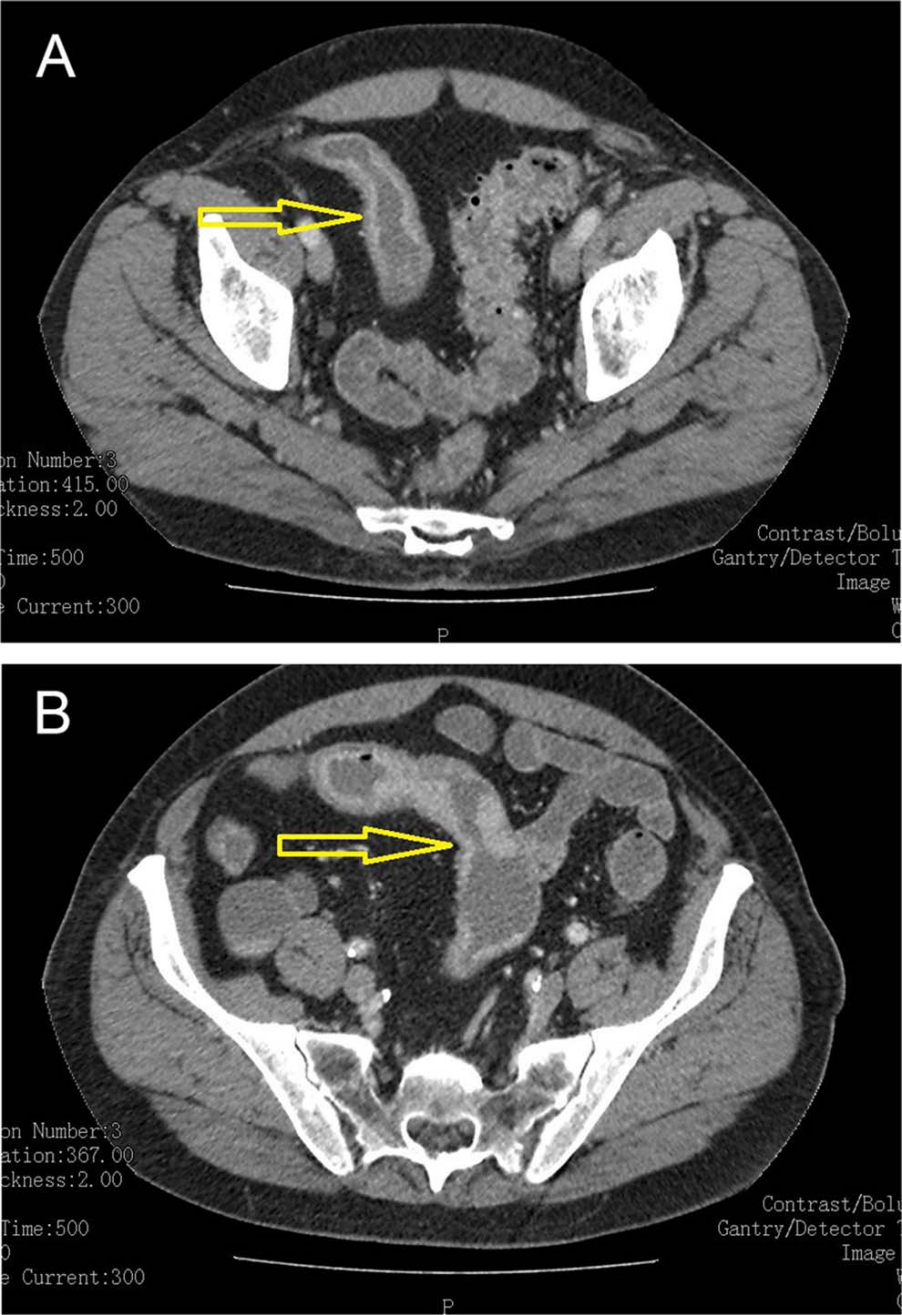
(A and B) Axial section of computed tomography enterography demonstrates wall thickening of several loops of the ileum (arrows).
